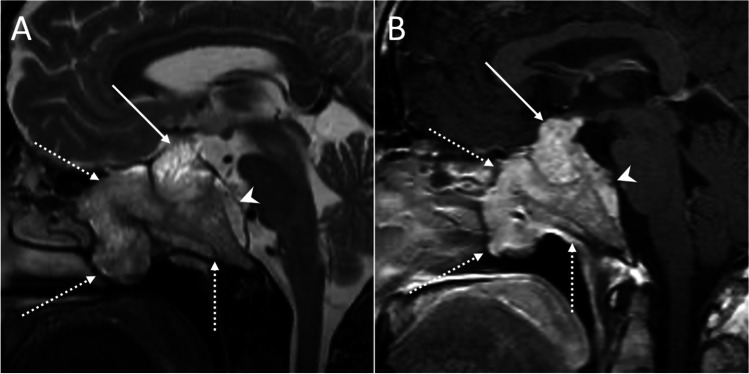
Invasive skull base pituitary adenoma. Sagittal T2- and contrast-enhanced T1-weighted images depict an intra- and suprasellar lesion (arrows) invading the adjacent central skull base (dotted arrows) and emerging to the retroclival epidural space (arrowheads)
